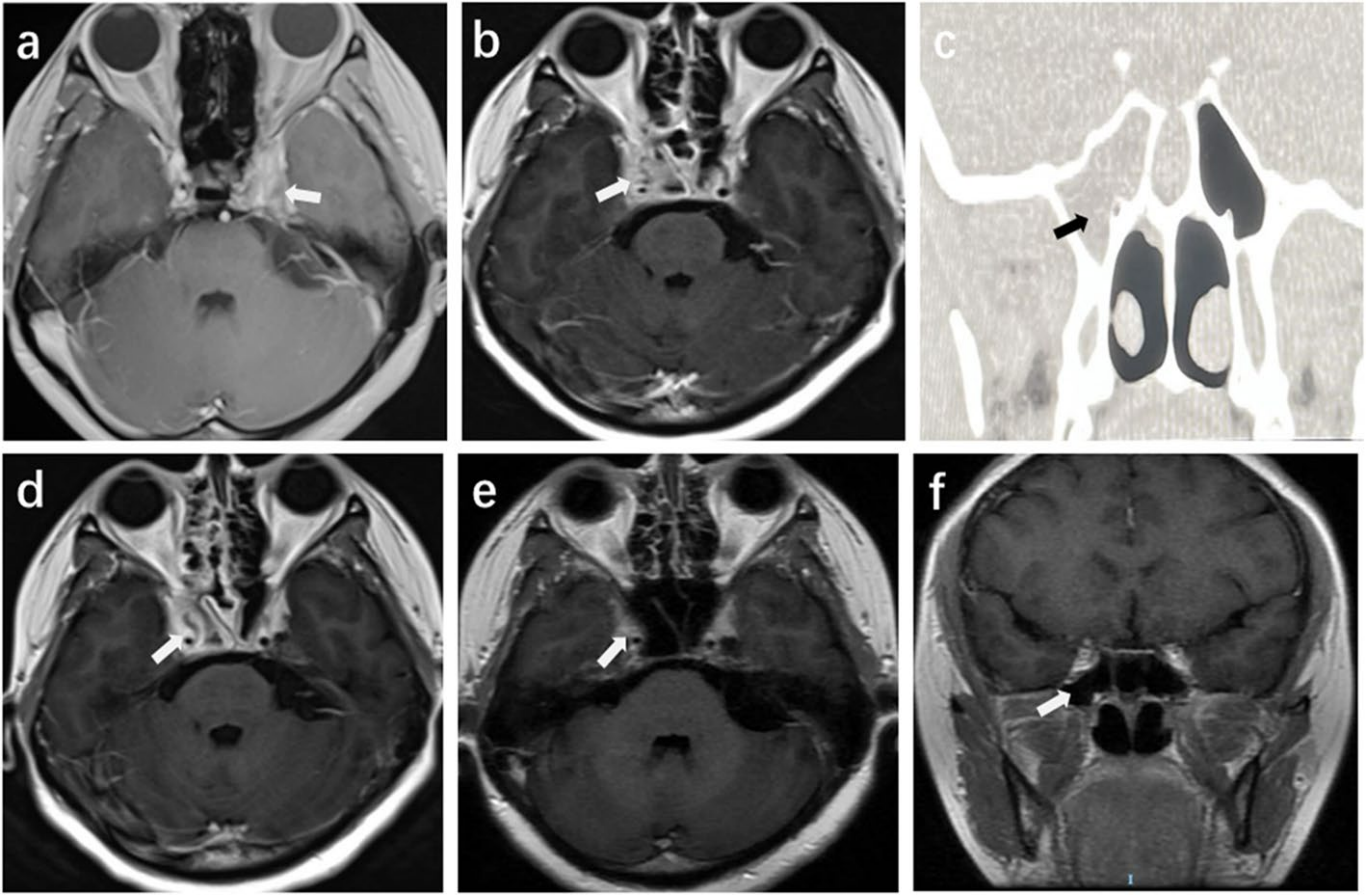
a Contrast-enhanced coronal T1 MRI of the first headache attack (left headache) on December 12, 2020. The left cavernous sinus was enlarged and widened. The abnormal tissue was strongly enhanced after the intravenous injection of the contrast material. b Contrast-enhanced coronal T1 MRI of the second headache attack (right headache) on January 10, 2021. The increased soft tissue shadows were present in the bilateral cavernous sinuses and were more pronounced on the right. c Axial sinus CT on January 14, 2021 shows inflammation of the right sphenoid sinus cavity. d One month after surgical and antibiotic therapy, MRI showed that the signal shadow of the cavernous sinus was less than before, although inflammation was still observed in the right. e-f: Two months after treatment, MRI showed a normal cavernous sinus and sphenoid sinus
